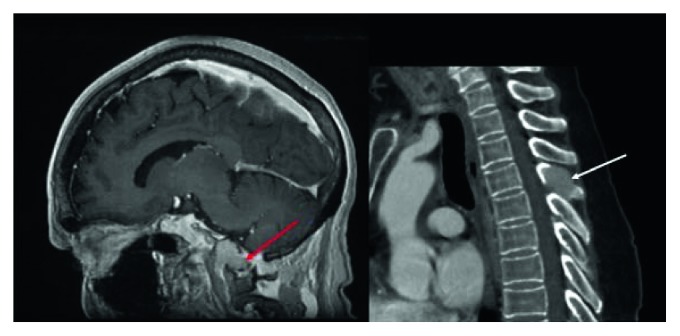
Magnetic resonance imaging (MRI) of the head and spine. MRI captured a 2.8 × 2.2 × 1.9-centimeter enhancing lytic mass centered in the left clivus and occipital condyle (red arrow). Additionally, an expansile soft tissue lesion was noted in the T4 spinous process (white arrow).
